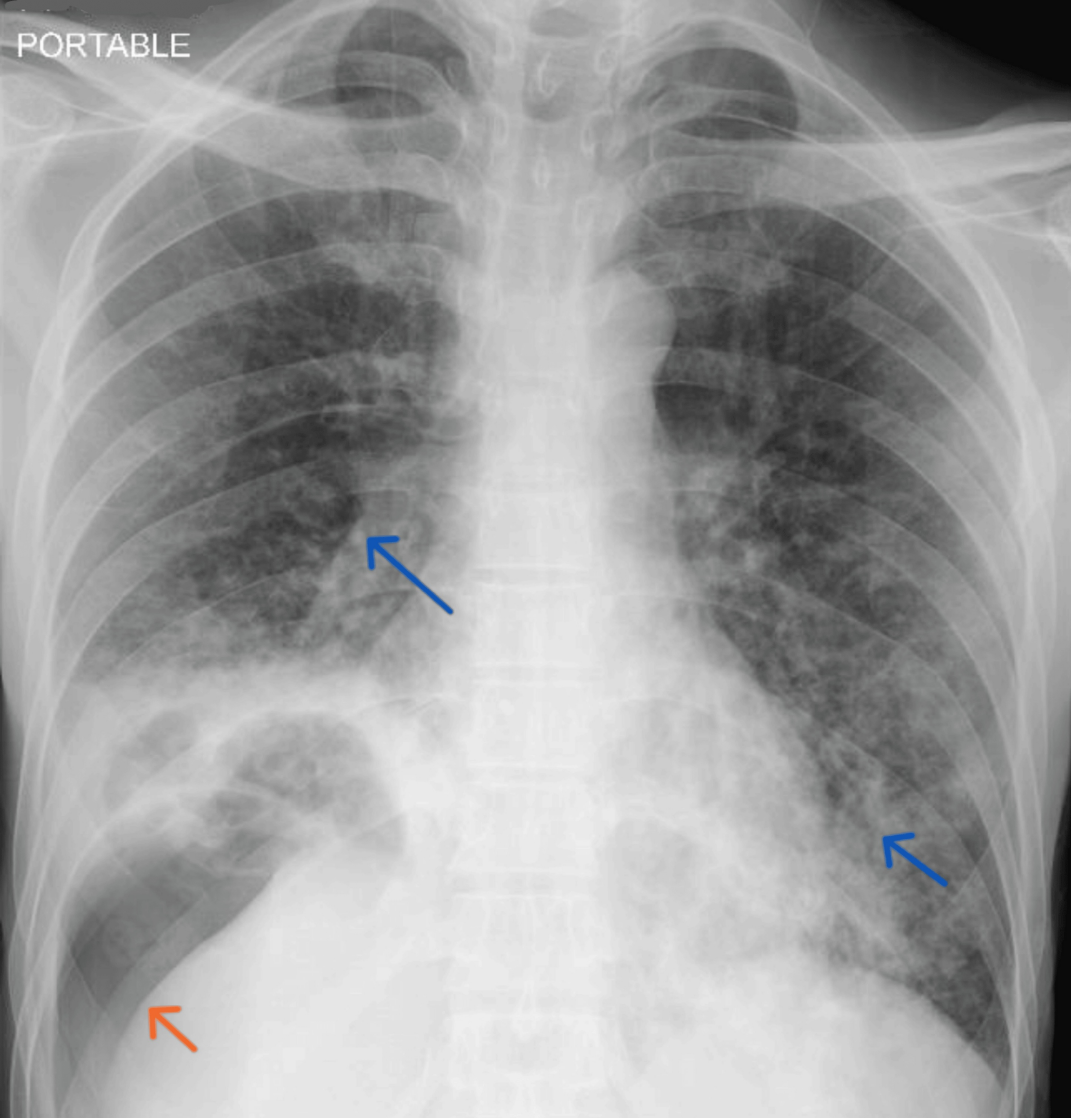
A portable bedside AP Chest X-ray demonstrates a dense consolidation with loculated air within the lower lobe of the right lung (orange arrow) and bilateral patchy infiltrates (blue arrow). AP: anteroposterior.
